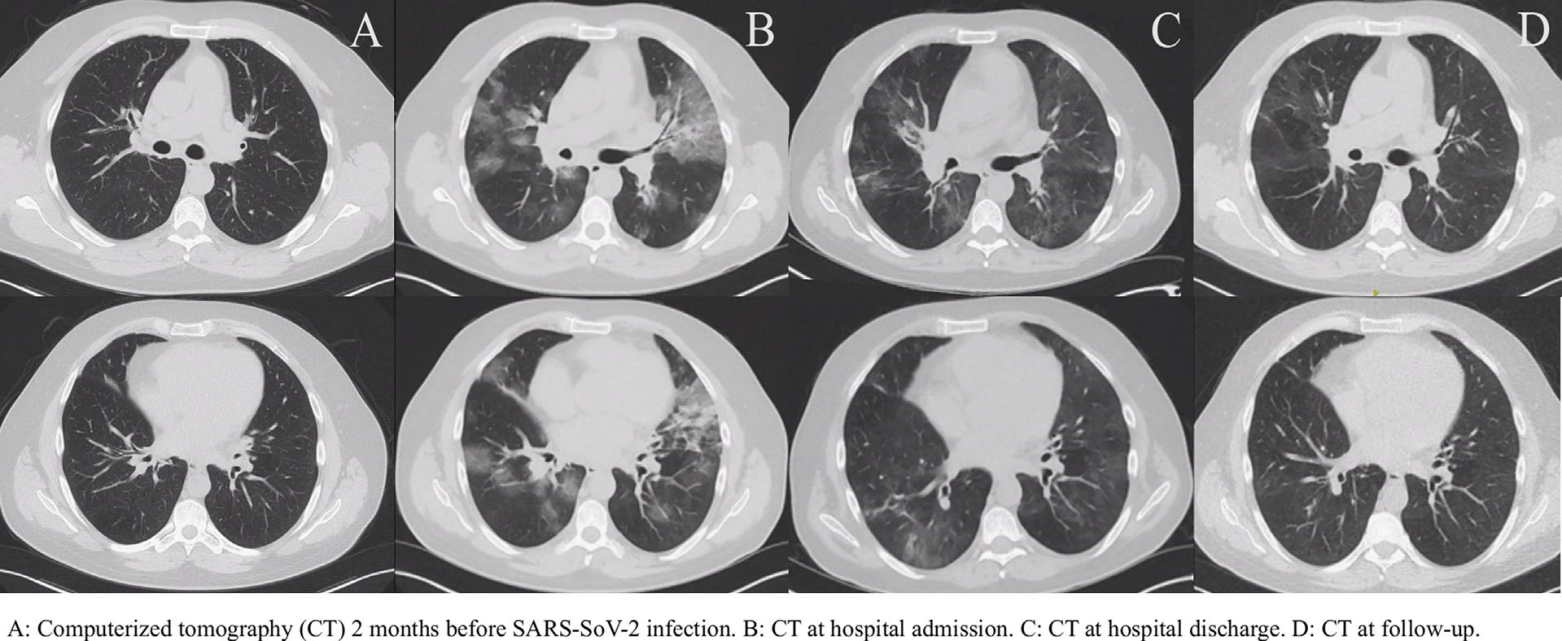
Complete resolution of the pulmonary infiltrates following recovery. (A) Computerized tomography (CT) 2 months before SARS-SoV-2 infection. (B) CT at hospital admission. (C) CT at hospital discharge. (D) CT at follow-up.
